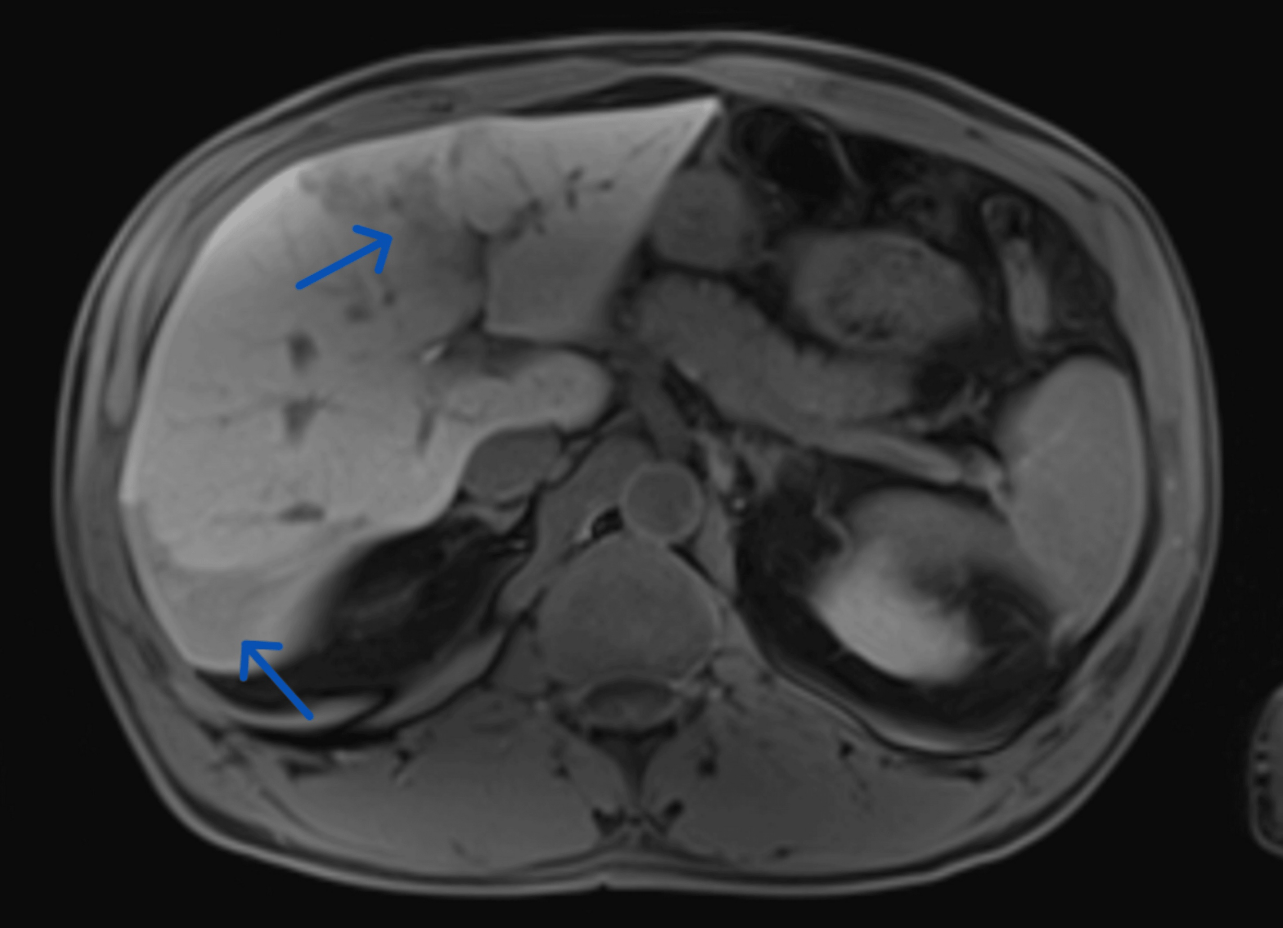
Abdominal MRI performed after first-line treatment with triclabendazole. The abdominal MRI shows an increase in the size of the pseudonodular hepatic lesions (arrows).
